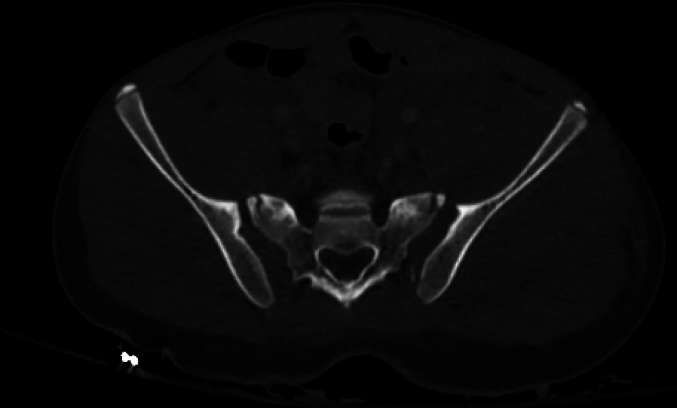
Axial cut from CT pelvis demonstrating bilateral SI joint dislocations in case 2.
